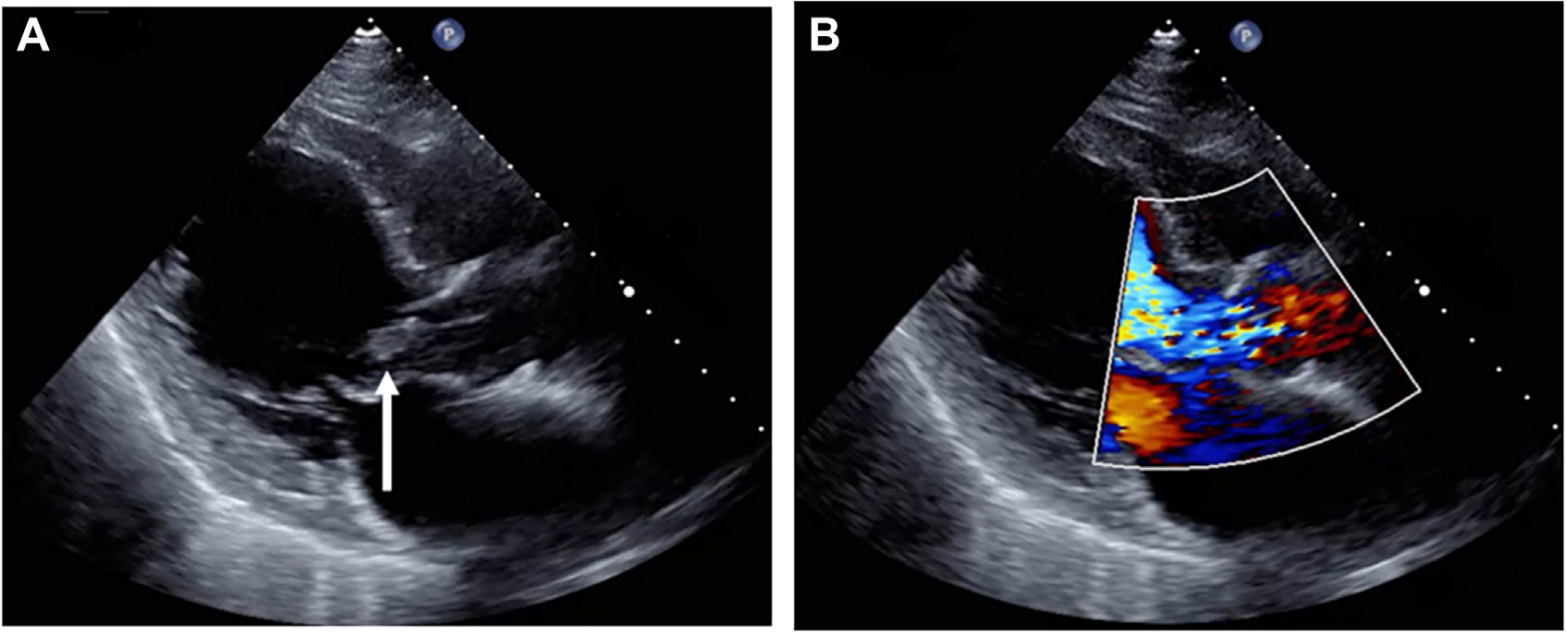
(A) The arrow points to vegetation on the aortic valve. (B) Transthoracic echocardiography demonstrated severe aortic regurgitation.
